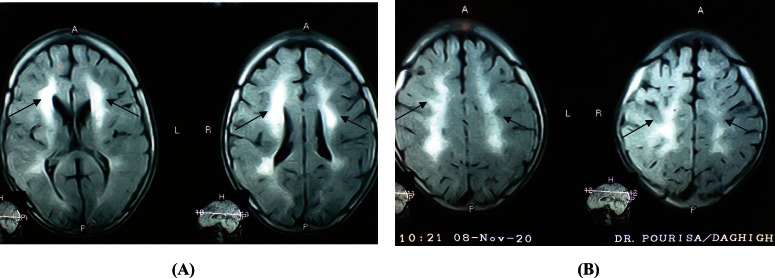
On brain magnetic resonance imaging (MRI), symmetrical confluent hypersignal areas of subcortical white matter were evident with decreased signal of both basal ganglia and pachygyria
